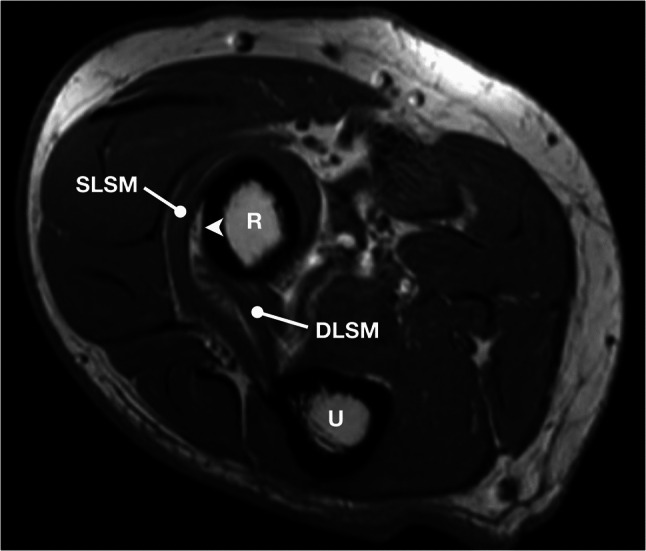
Right elbow MRI showing the bare area (arrowhead) with a direct contact with the deep branch of the radial nerve. DLSM = deep layer of the supinator muscle; R = radius; SLSM = superficial layer of the supinator muscle; U = ulna
